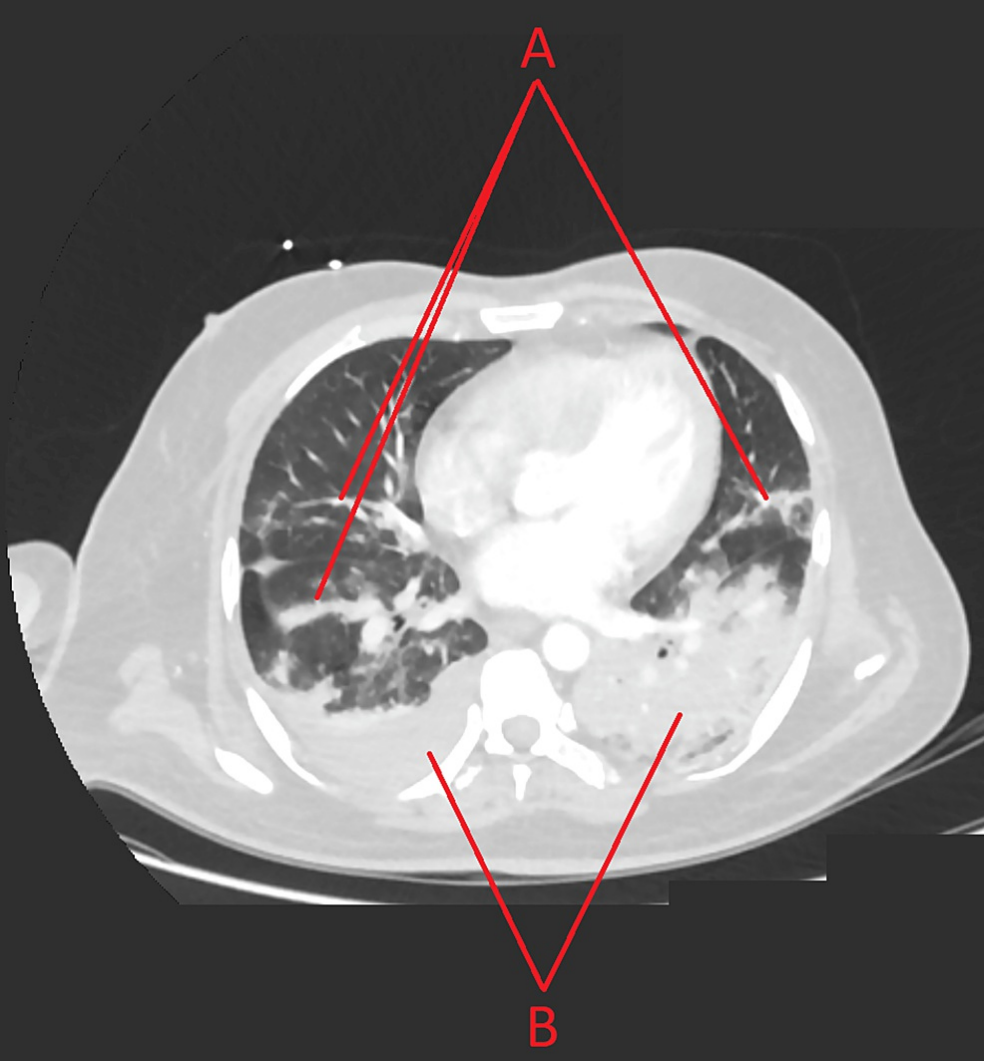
A CT pulmonary angiography. A) Bilateral patchy consolidation denoting an active infection. B) Mild pleural effusion
